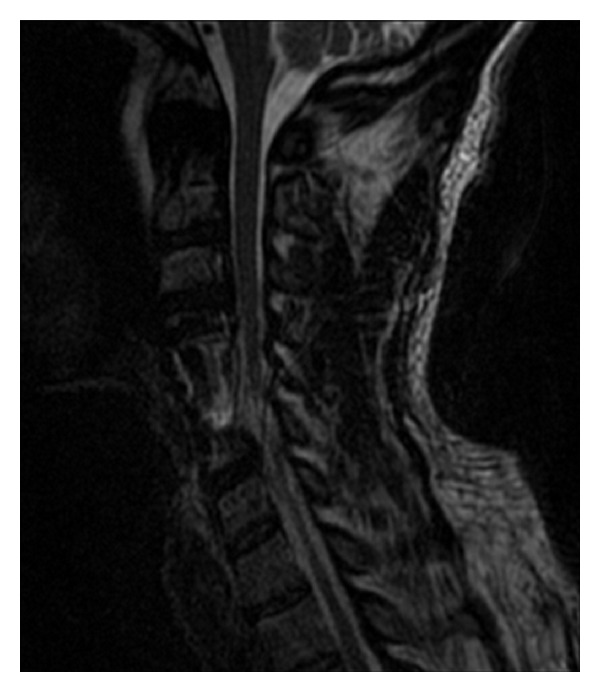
Day 3 postoperative MRI demonstrates more clearly the spinal cord edema intrinsic to the cord—a “white cord syndrome.”
